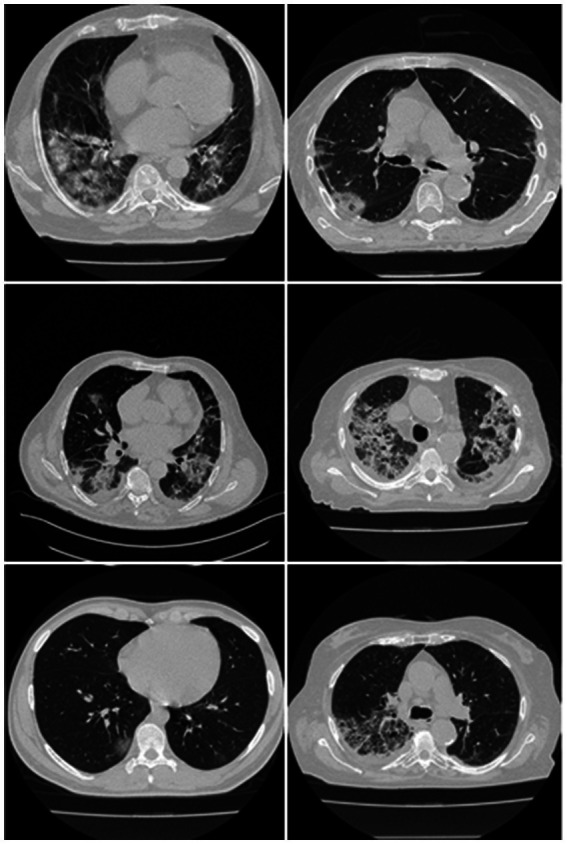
Raw CT images from the Croatian dataset.
